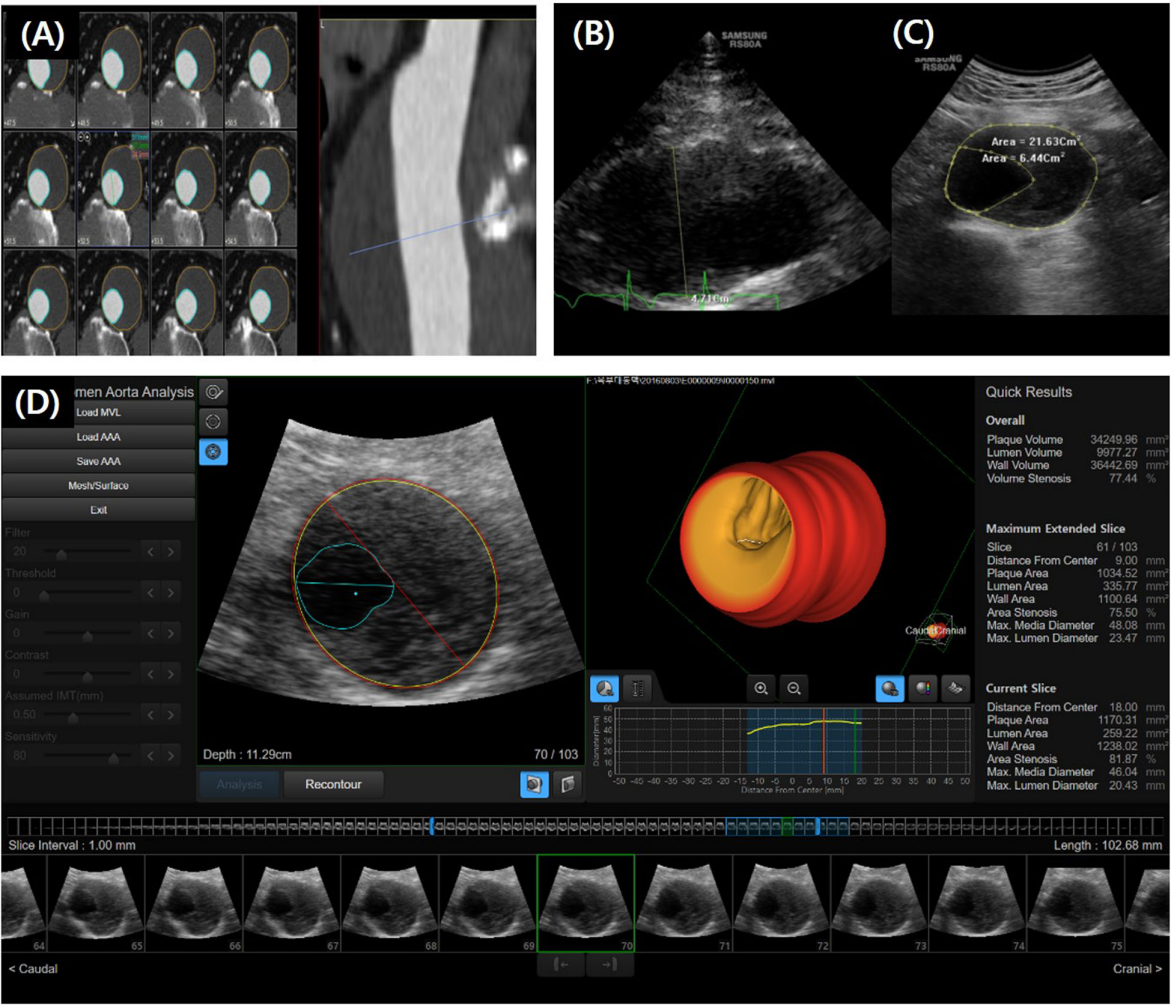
A representative case comparing the analysis of aortic diameter, area, and thrombus volume by different tests in abdominal aortic aneurysm: (a) aorta computed tomography, (b) two-dimensional (2-D) longitudinal, (c) 2-D axial images, and (d) three-dimensional (3-D) automated software analysis. The yellow circle marks the intima-lumen boundary; the red circle, the media-adventitia interface; and blue circle, the thrombus boundary in the automated 3-D ultrasonographic software analysis in D. The red line represents the maximum diameter of the vessel, and the blue line represents the maximum diameter of the non-thrombosed lumen
